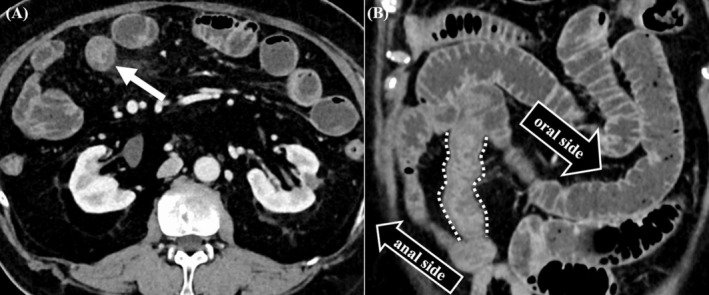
Contrast‐enhanced CT findings on admission. (A) Axial CT image demonstrating a transition point (arrow) in the small bowel. (B) The affected bowel segment (outlined with a dashed line) shows segmental mural thickening and luminal narrowing. The distal (anal side) bowel is collapsed, whereas the proximal (oral side) bowel is dilated with a small bowel feces sign. These findings mimic mechanical small bowel obstruction.
